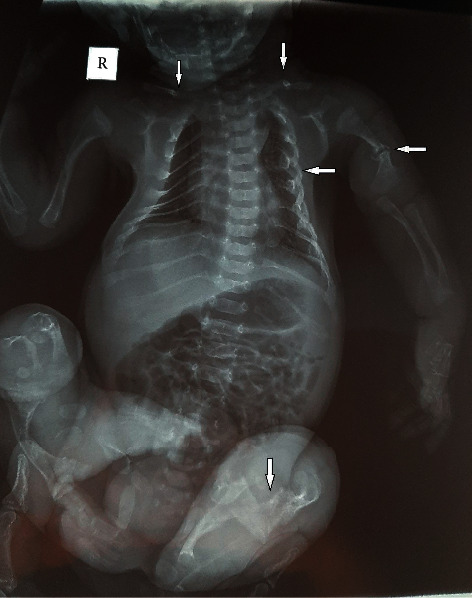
Plain radiograph showing fractures of bilateral clavicles, left humeral diaphysis, bilateral lower limbs, and bilateral ribs which were marked in the left hemithorax (fracture lines shown by arrowhead).
